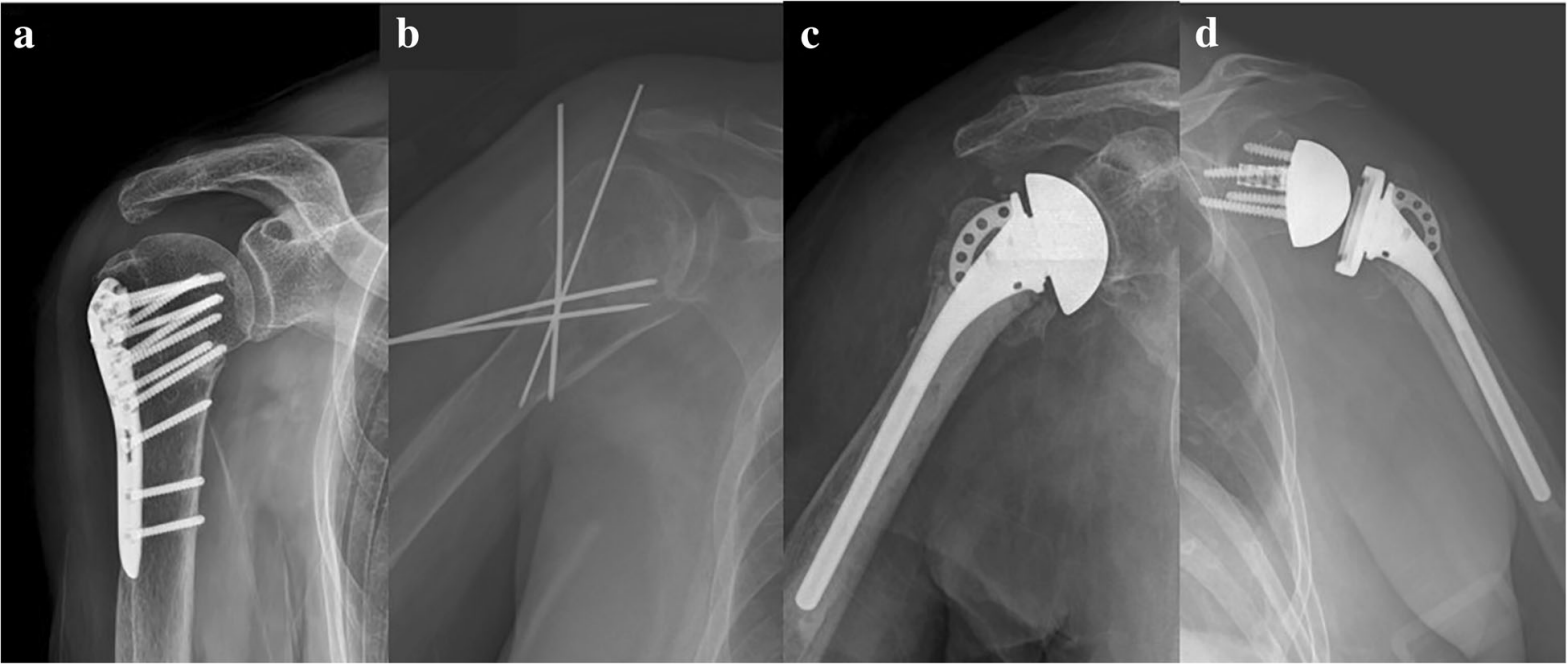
Plain radiographs demonstrating types of surgical treatment of proximal humeral fractures: (a) open reduction and internal fixation (b) closed reduction and internal fixation (c) hemiarthroplasty (d) reverse shoulder arthroplasty. All surgeries took place at Hanyang University Medical Centre, and patients gave informed consent for the publication of their radiographs
